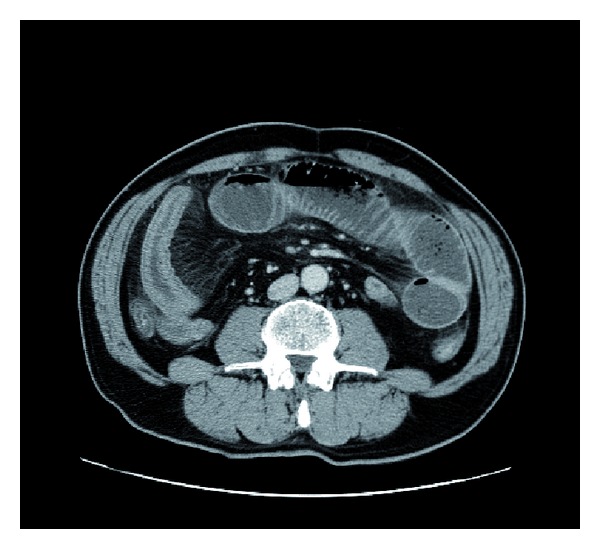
Contrast-enhanced abdominal CT findings demonstrated small intestinal dilatation with wall thickening and contrast enhancement.
